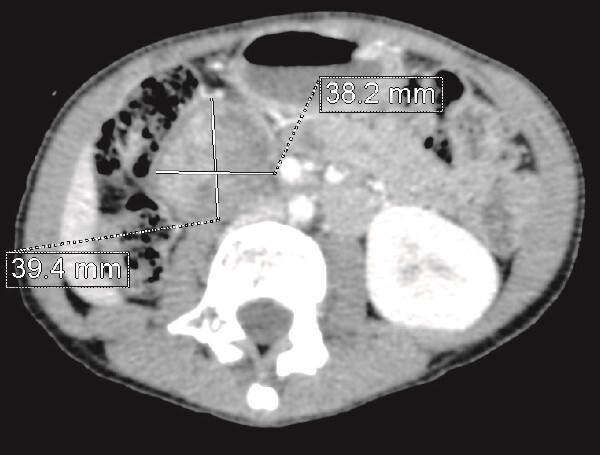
CT scan evaluation after chemotherapy: Regression in size of the right kidney mass with an estimated response of 75%.
